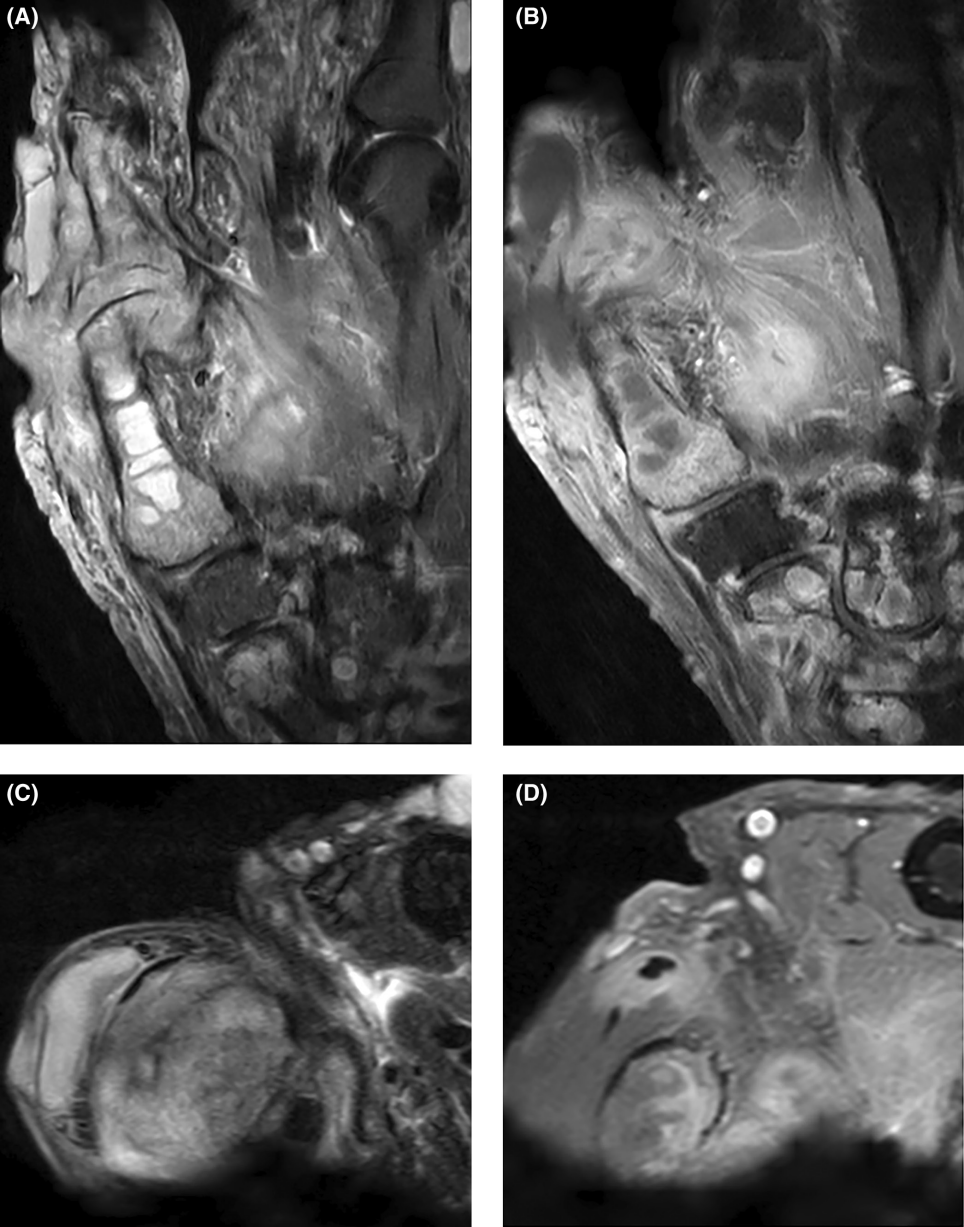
MRI right thumb *. (A) Coronal STIR without contrast (top left image). (B) Coronal T1 with contrast (top right image). (C) Axial T2 without contrast (bottom left image). (D) Axial T1 with contrast (bottom right image). (E) *note there is significant motion artifact.
